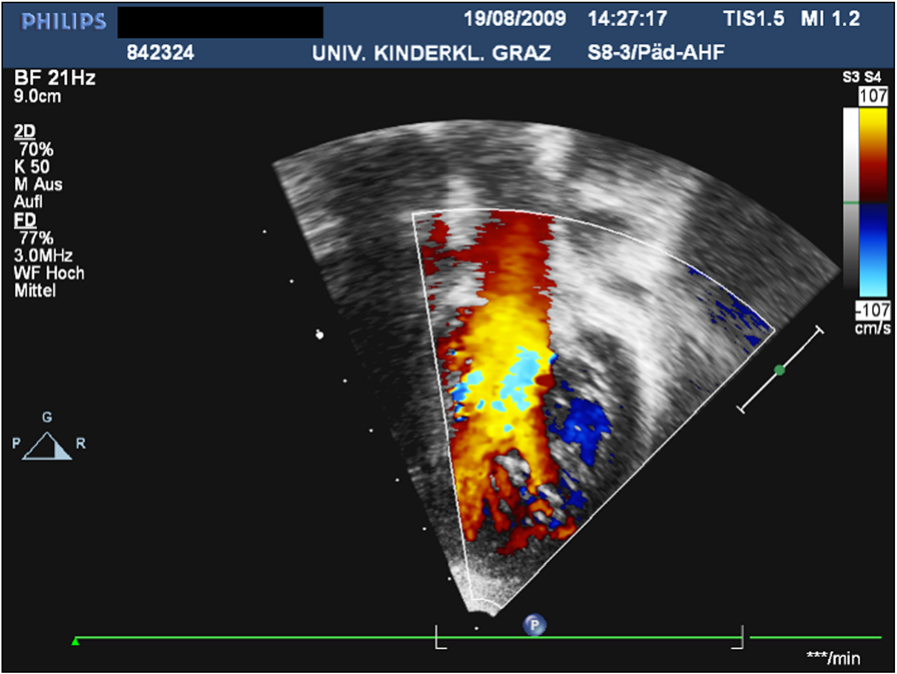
Using colour-Doppler-sonography the intertrabecular recesses are perfused from the ventricular cavity.
